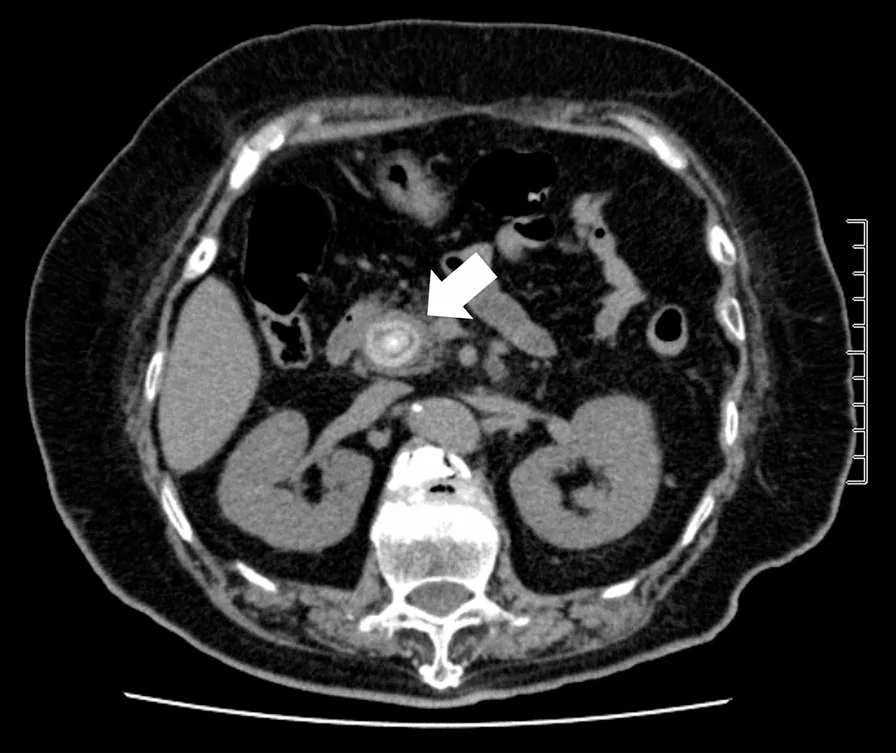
Abdominal CT scan at the first visit revealing dilatation of common bile duct and large choledocholithiasis (arrow)
